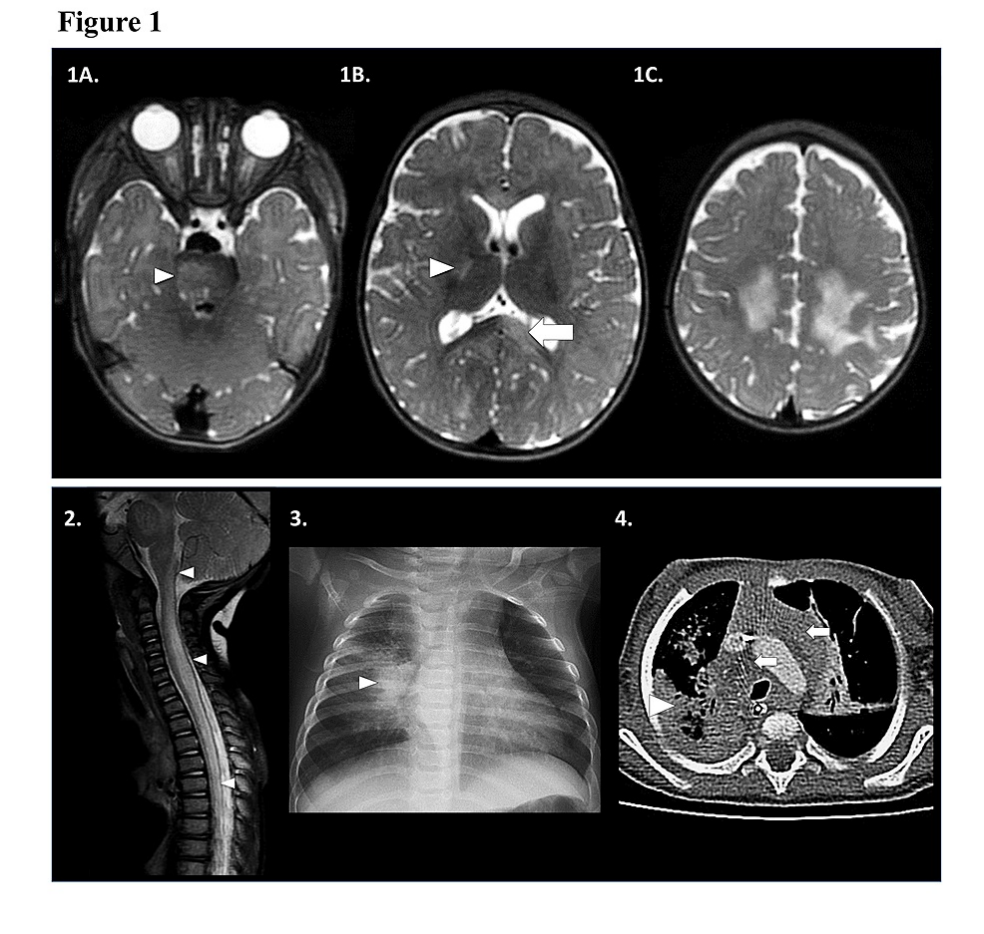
Neuroimaging and chest imaging 1) MRI brain, transaxial T2-weighted images showing multifocal T2-hyperintense foci in the: (A) right pons [white arrowhead]; (B) posterior limb of the right internal capsule [white arrowhead] and left splenium of the corpus callosum [white arrow]; (C) bilateral centrum semiovale and frontoparietal subcortical white matter. Other MRI sequences not shown revealed no associated reduced diffusivity, blood products, or contrast enhancement. 2) MRI spine, sagittal T2-weighted image showing extensive bilateral intramedullary T2-hyperintense edema and swelling along the cervicomedullary junction and throughout the cervical, upper and visualized mid thoracic spinal cord. No associated contrast enhancement was present on other MRI sequences. 3) Frontal chest radiograph: right perihilar opacity and hilar lymphadenopathy [white arrowhead]. Additional right upper, and left lower pneumonia and left hilar lymphadenopathy present. 4) Transaxial contrast-enhanced CT chest: dense necrotic right lower lobe [white arrowhead] with additional right middle and left upper lobe pneumonia, and bilateral bulky mediastinal lymphadenopathy [white arrows]. There was no enhancement or reduced diffusion.
